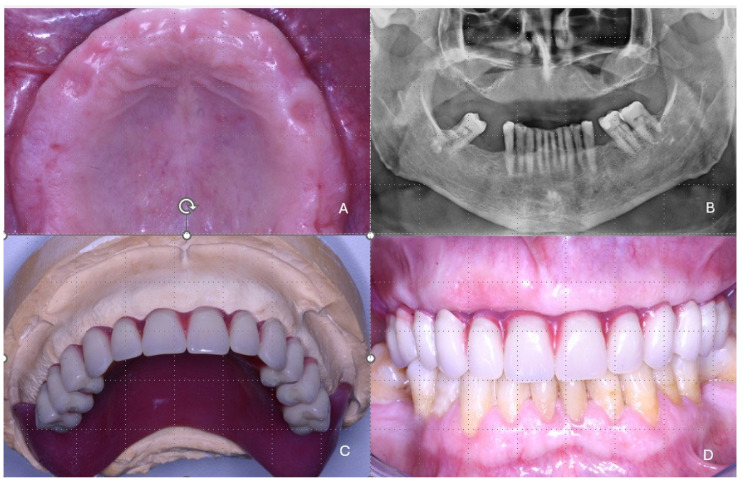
(A) Initial clinical situation, (B) Initial radiographic situation, (C) Diagnostic set-up, (D) Try-in of the diagnostic set-up to the patient.
